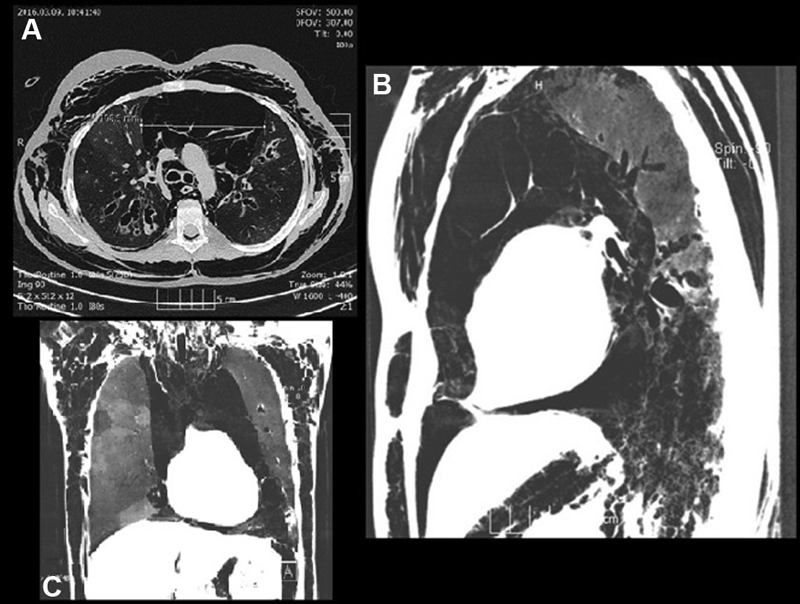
Computed tomography scan (in the level of bifurcation) depicted an extensive subcutaneous emphysema and a huge pneumomediastinum (diameter: 10.8 cm): (
